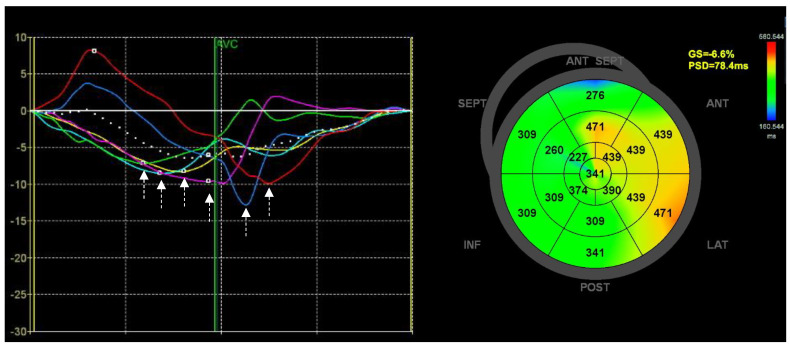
Mechanical dispersion. The figure shows an example of strain analysis, for assessing mechanical dispersion, in a patient with ischemic cardiomyopathy and ICD who experienced appropriate therapy after implantation. On the left, segmental strain profiles from the apical 4–chamber view are shown, with white arrows highlighting a heterogenous timing of peak strain values for the segments (each colored curve represents a single segment). On the right, a bulls–eye plot of time–to–peak strain is shown for all segments, and mechanical dispersion is provided as the peak systolic dispersion (PSD), calculated as the standard deviation of the time–to–peak of the segmental strain.
